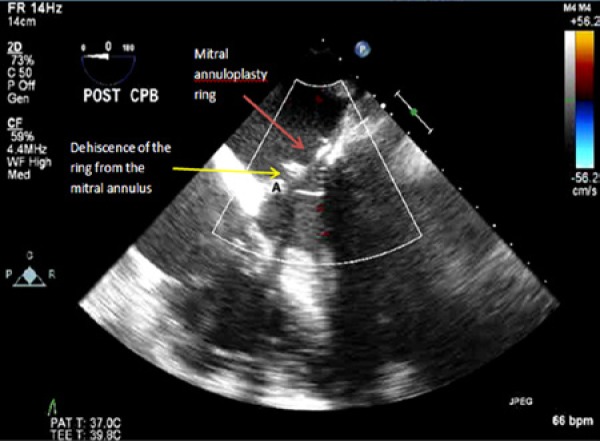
Two-dimensional transesophageal echocardiography midesophageal 4-chamber view showing dehiscence of the annuloplasty ring. Abbreviation: A, mitral annulus.
